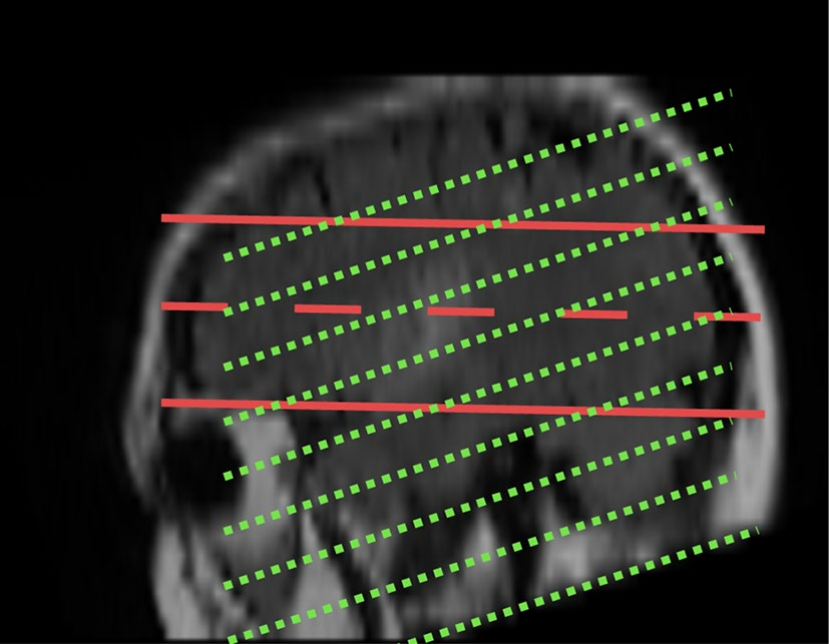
Sagittal plane of the human head showing the plane of imaging in EM and MRI slices. The plane of the original MRI slices is depicted with green dotted lines, and the two solid red lines show the imaging volume of the array. The dotted red line indicates the axial center of the antenna array.
